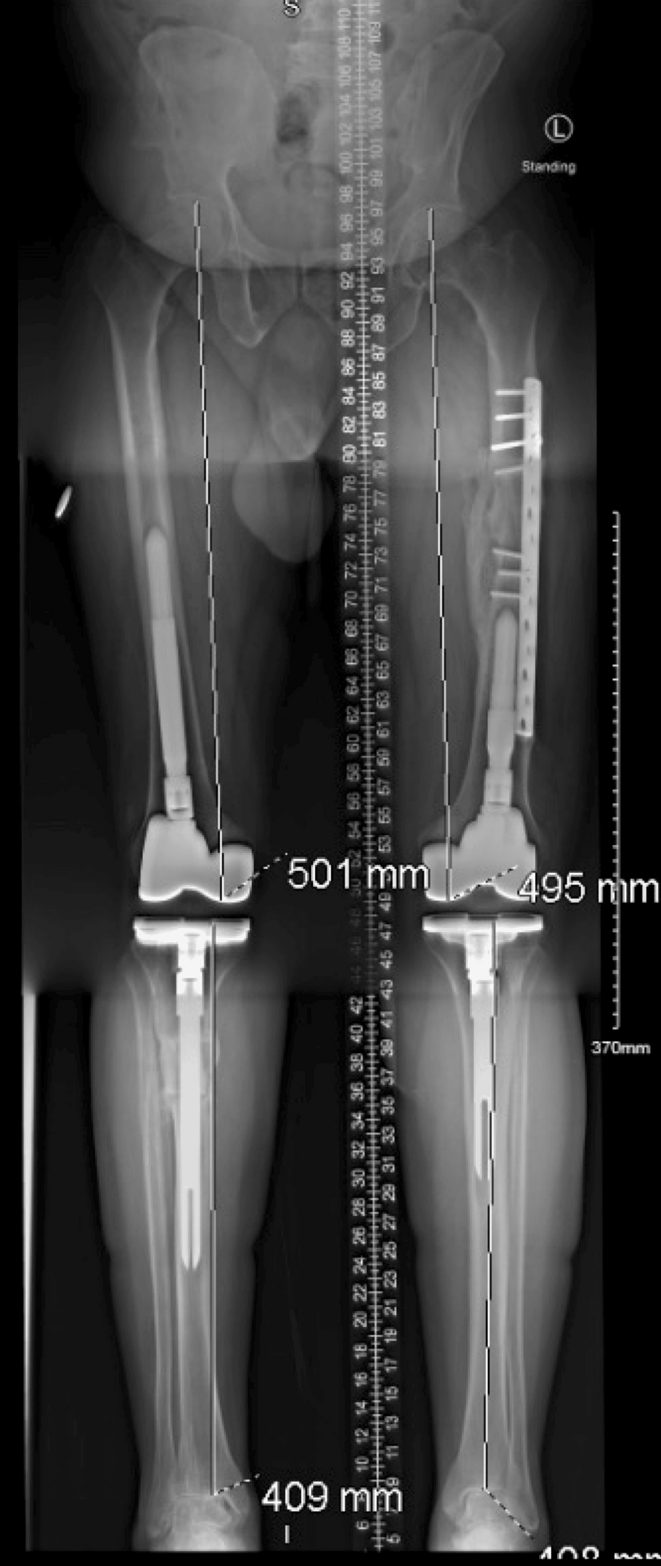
Full-length standing radiography showing mechanical restoration after total knee arthroplasty
